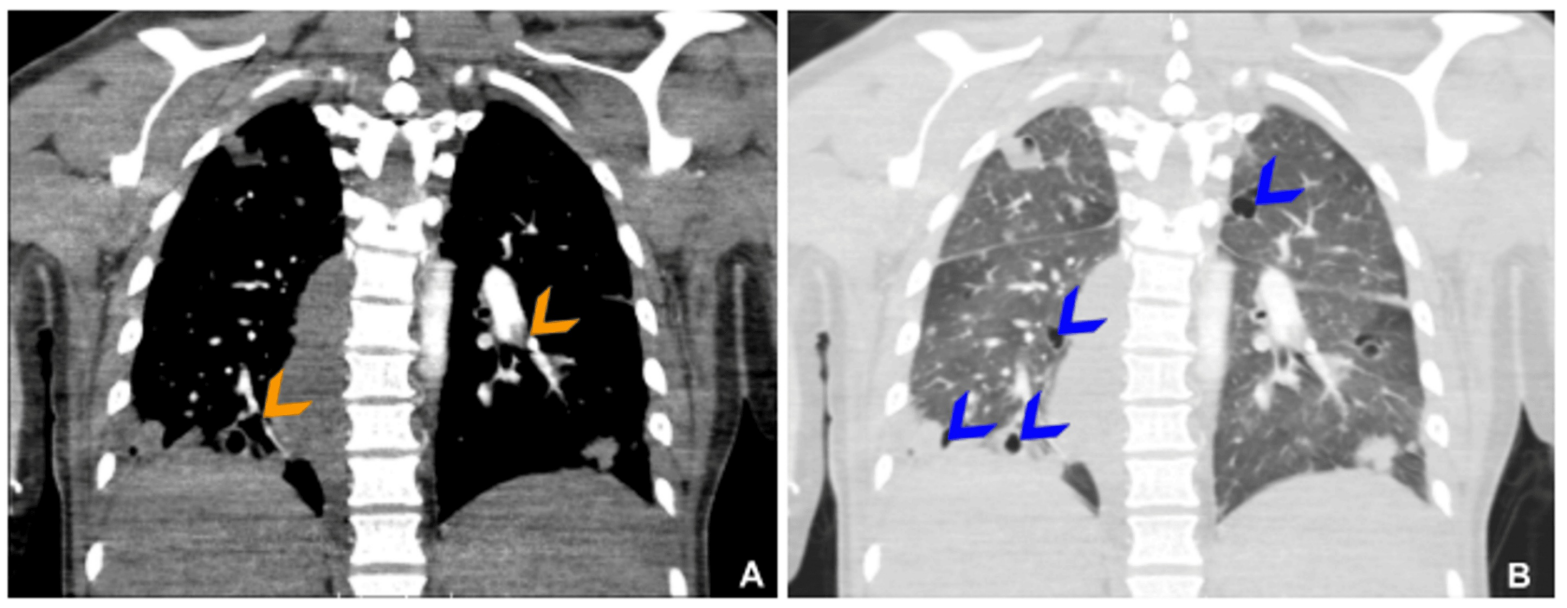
CT findings: bilateral pulmonary emboli and necrotic airspace opacities. A coronal chest CT scan revealed bilateral segmental pulmonary emboli (orange arrows, image A) without evidence of central embolus or overt right heart strain. Numerous nodular airspace opacities were seen bilaterally (blue arrows, image B), some demonstrating cystic and necrotic components, compatible with septic emboli.
